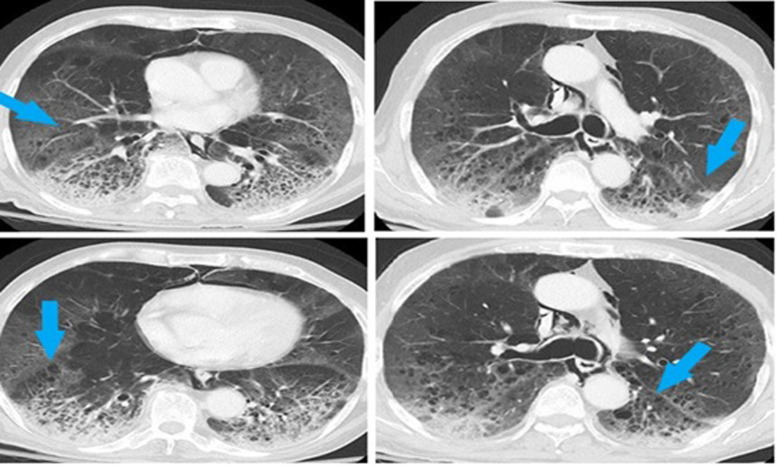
CT chest showing ground glass haziness with pulmonary cysts.
